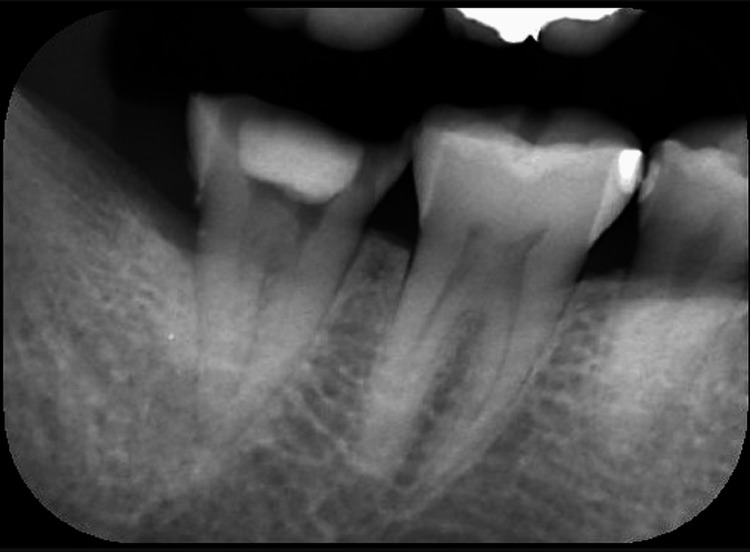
Intraoral radiograph In the right mandibular second molar, a root canal treatment was in progress, but there were no findings that would cause throbbing pain.
